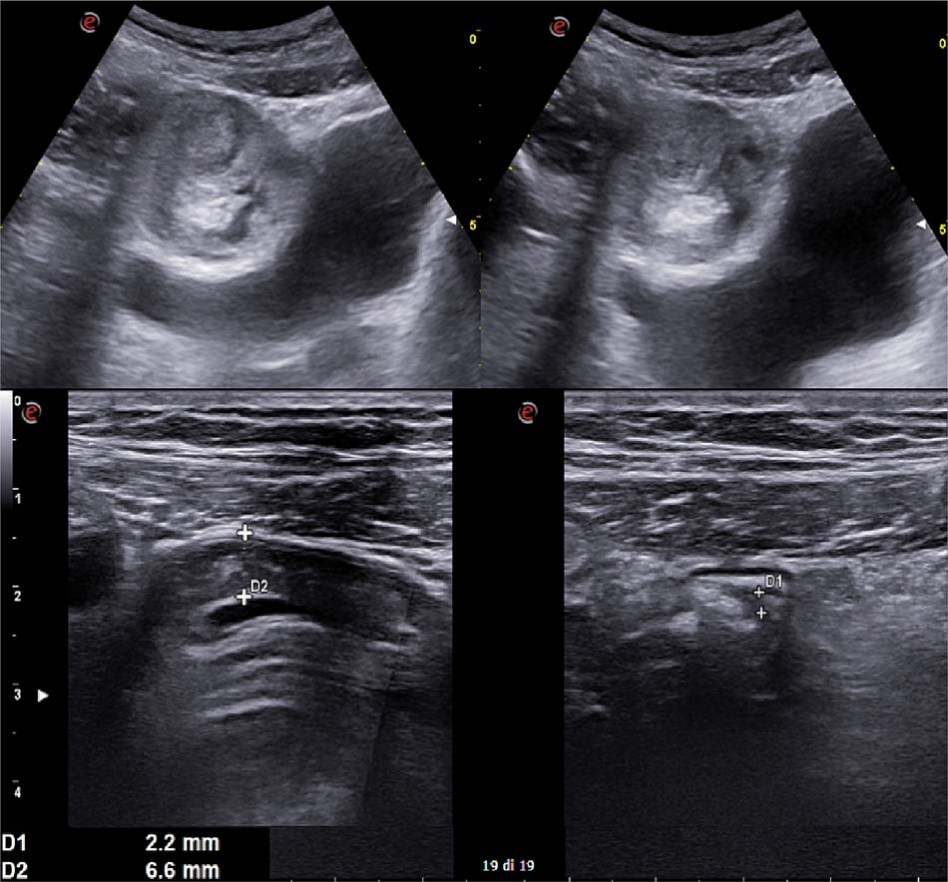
Abdomen ultrasound image displaying visceral distension with a cockade appearance consistent with an inflammatory condition of the cecum and the ileocecal site, showing wall thickening of 6 up to 10 mm and free fluid near the cecum.
